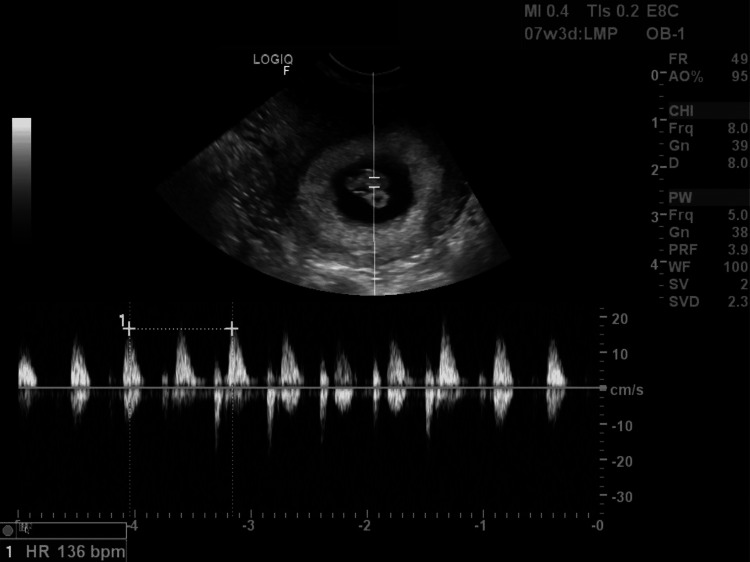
A transvaginal ultrasound of the fetus with present cardiac activity at 7w3d in what is suspected to be a right rudimentary uterine horn.
